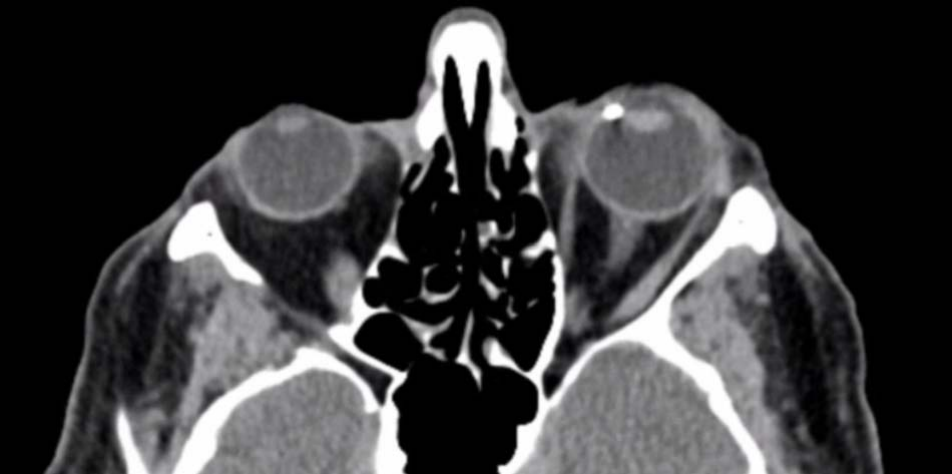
Axial CT scan of the eyes showing a 4-mm dense foreign body in the angle of the anterior chamber of the left eye, located nasally.
